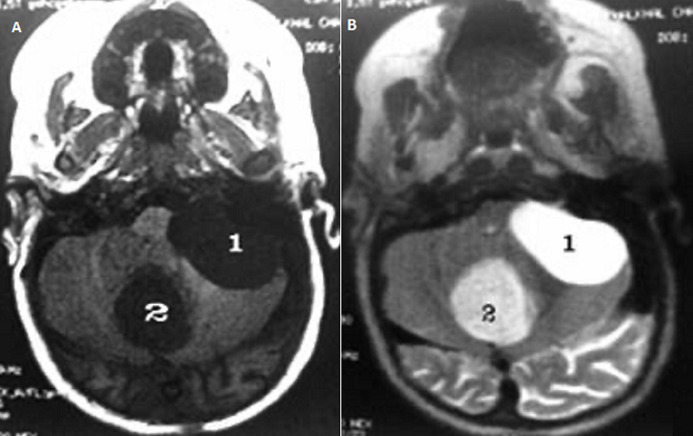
A) cerebral MRI in axial section performed in T1; B) cerebral MRI in axial section performed in T2 mode showing the arachnoid cyst of the left cerebellopontine angle (1) in hypo-signal T1 (A) and in hyper-signal T2 (B) and the vermian dermoid cyst (2) in hypo-signal T1 (A) and slightly in hyper-signal T2 (B)
